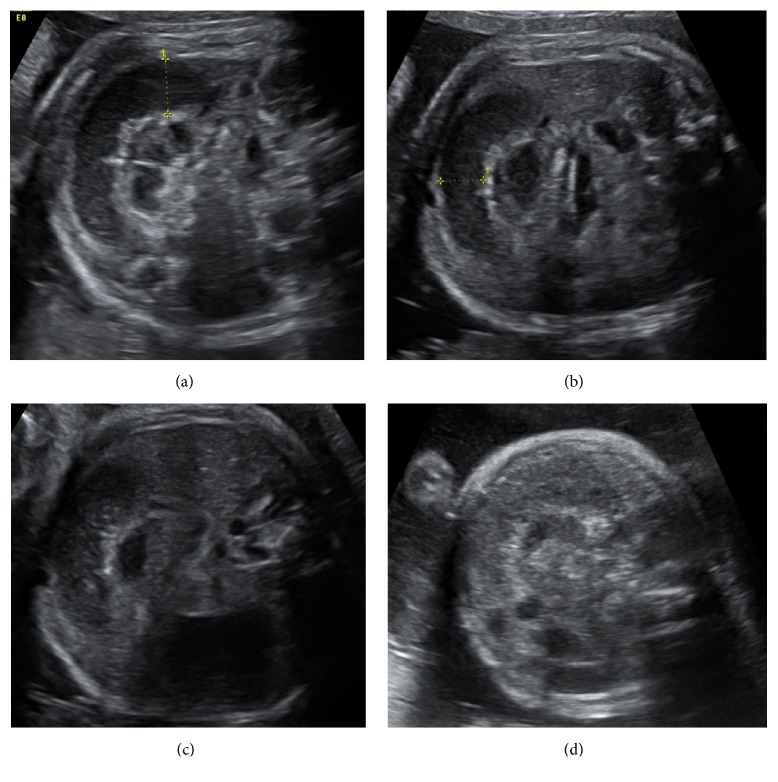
Ultrasound images showing progressive changes within the fetal bowel over time. (a) At 31^+6^ weeks, echolucent bowel contents with a diameter of 14 mm. (b) At 31^+6^ weeks, a loss of clear bowel wall border. (c) At 32 weeks, increasing echogenic particles within the bowel lumen. (d) At 32^+1^ weeks, bowel contents appear echogenic.
